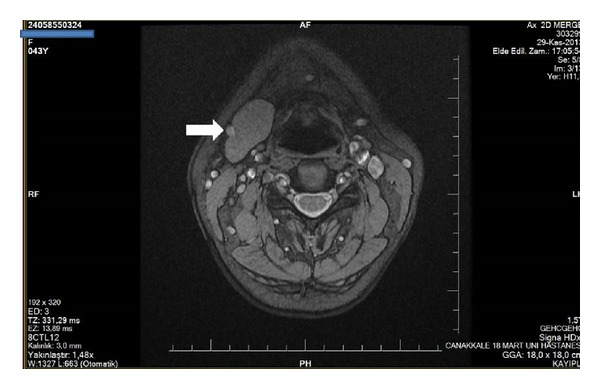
Magnetic resonance image axial sections demonstrate absence of left submandibular gland in the second case. White arrow shows the right submandibular gland.
